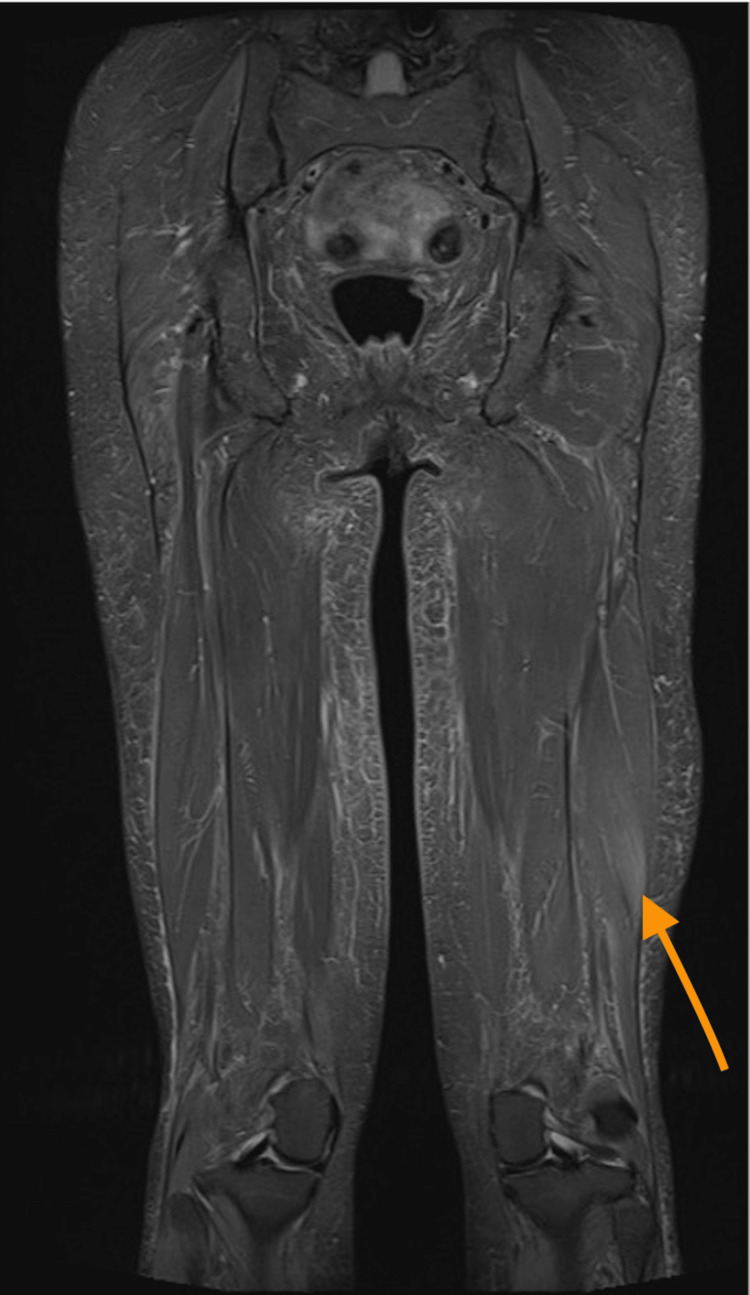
Coronal-section MRI (STIR sequence) of both thighs demonstrating localized myositis in the biceps femoris on the left side STIR: Short tau inversion recovery
